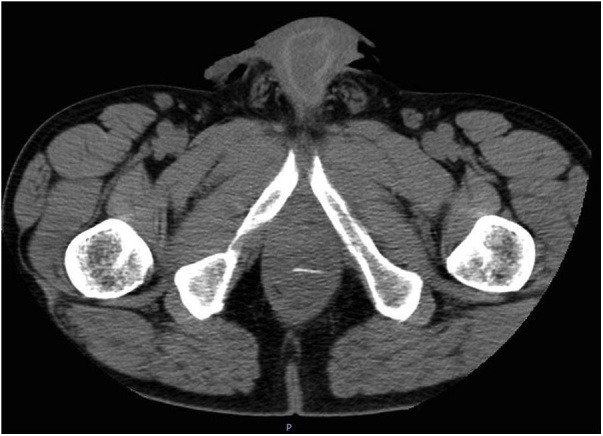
Pelvic computerized tomography (CT) revealed a sharp radiopaque image on the rectum.
